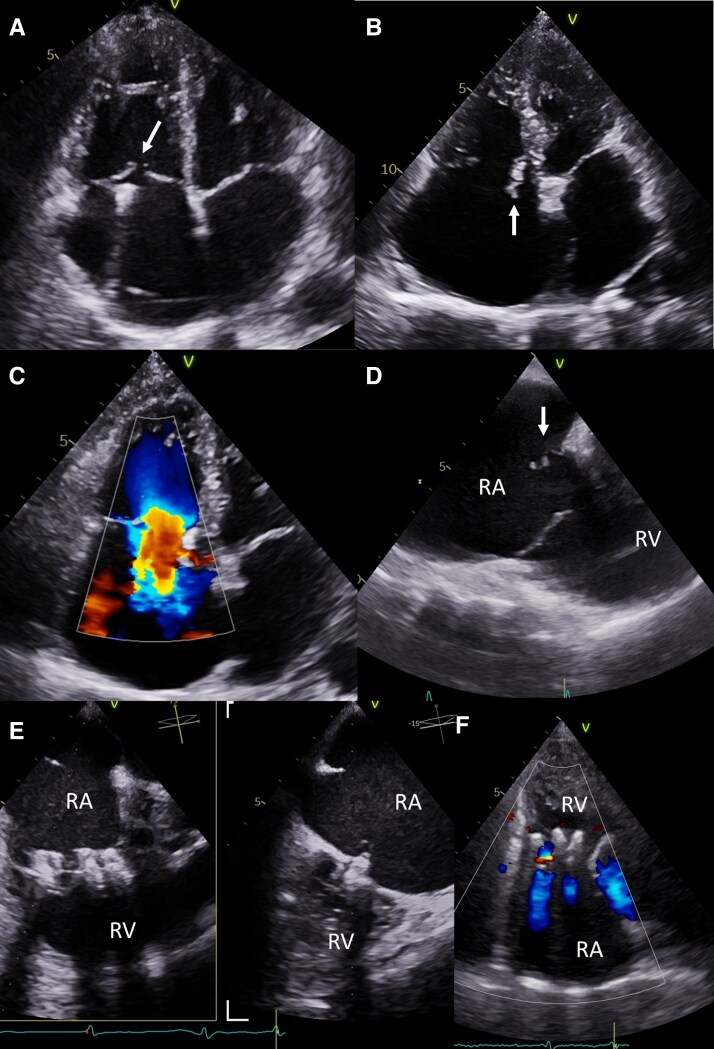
Patient 1 *(A)* Significant TR at baseline-before TLE, with ‘coaptation gap’ marked by arrow. No signs of the flail leaflet. *(B)* Flail septal leaflet of TV marked by arrow in TTE. *(C)* Torrential TR in colour Doppler. *(D)* Flail septal leaflet of TV is marked by an arrow in TEE. *(E)* Tricuspid valve after implantation of three PASCAL Ace devices. *(F)* Maintained TR reduction at follow-up visit. RA—right atrium; RV—right ventricle.
